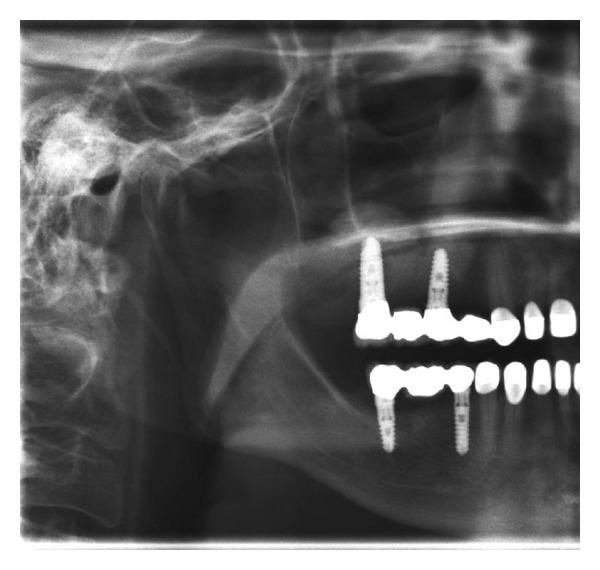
Follow-up panoramic X-ray after completion of implant insertion and prosthetic treatment 12 months following tHUCSL-Intralift.
